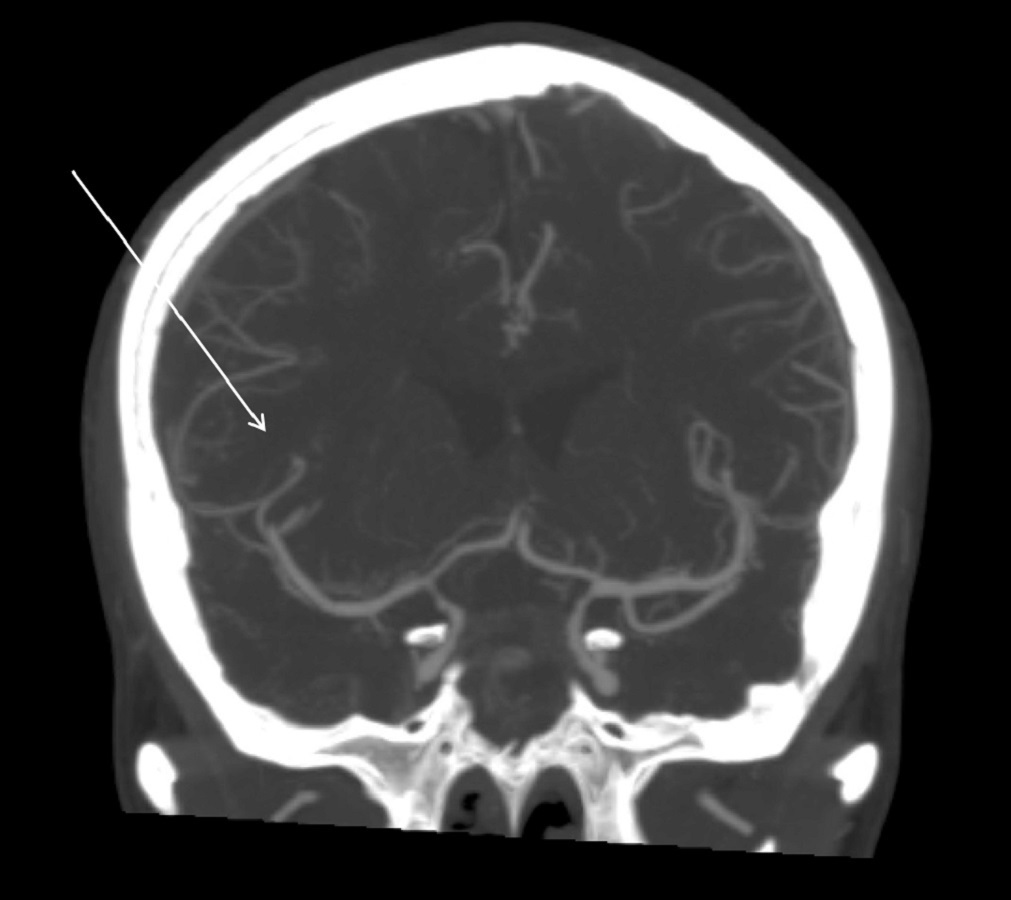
Computed tomography (CT) arteriography revealing significant vasospasm in the bilateral distal internal carotid arteries and distal basilar artery.
